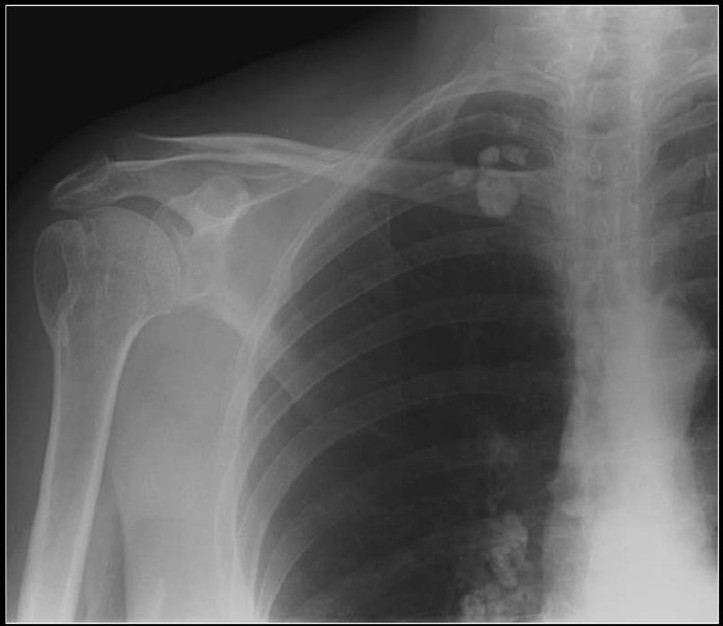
A radiograph taken for the right shoulder shows old healed granulomatous disease at the right apex of the lung associated with calcified right hilar lymph nodes
